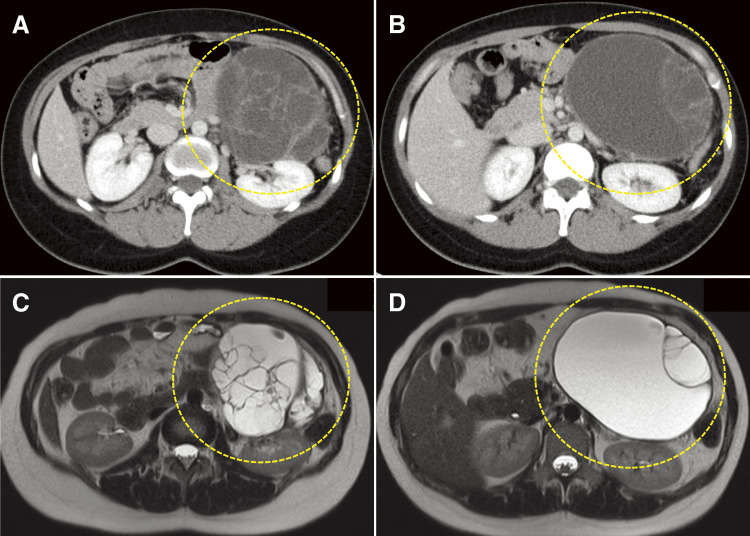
Imaging findings at the time of initial examination: (A, B) CECT. A multifocal cystic mass with a length of 130 mm in the pancreatic tail with multiple septa and a cyst appearance (dotted line) consistent with a pancreatic MCN. (C, D) T2-weighted magnetic resonance images. The cyst content had signal intensities akin to those of water. Although some of the septal walls appear thick, no mural nodules are observed. CECT, contrast-enhanced computed tomography; MCN, mucinous cystic neoplasm
